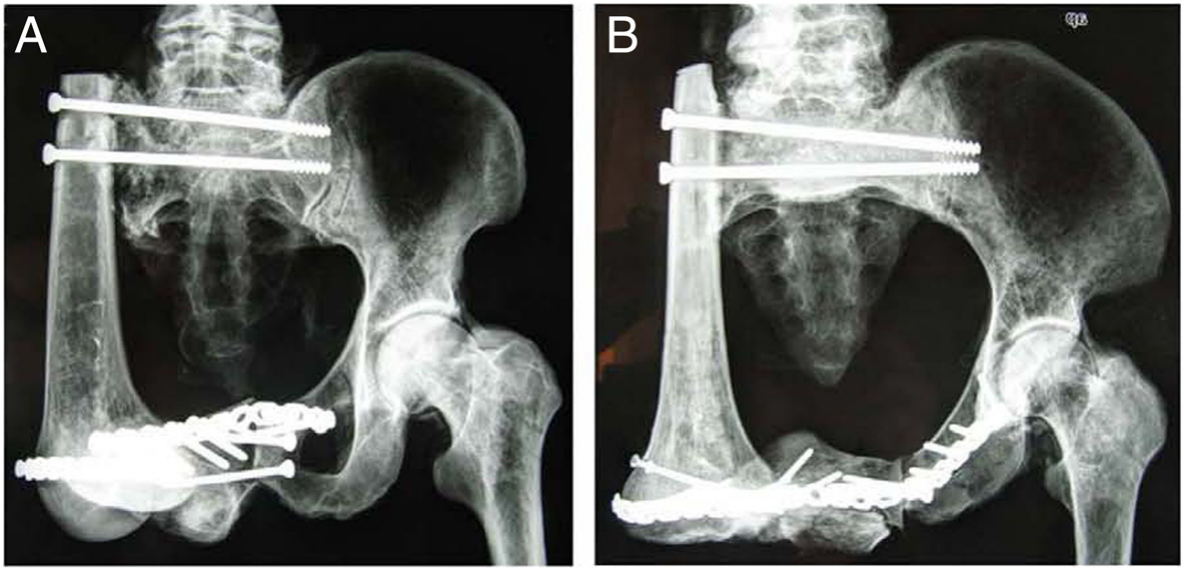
Radiographs of the reconstructed pelvic ring. Hemipelvic defects were stabilized with a screw-plate system. (A) Pelvis reconstructed with femoral autograft. (B) Pelvis reconstructed with tibial autograft.
